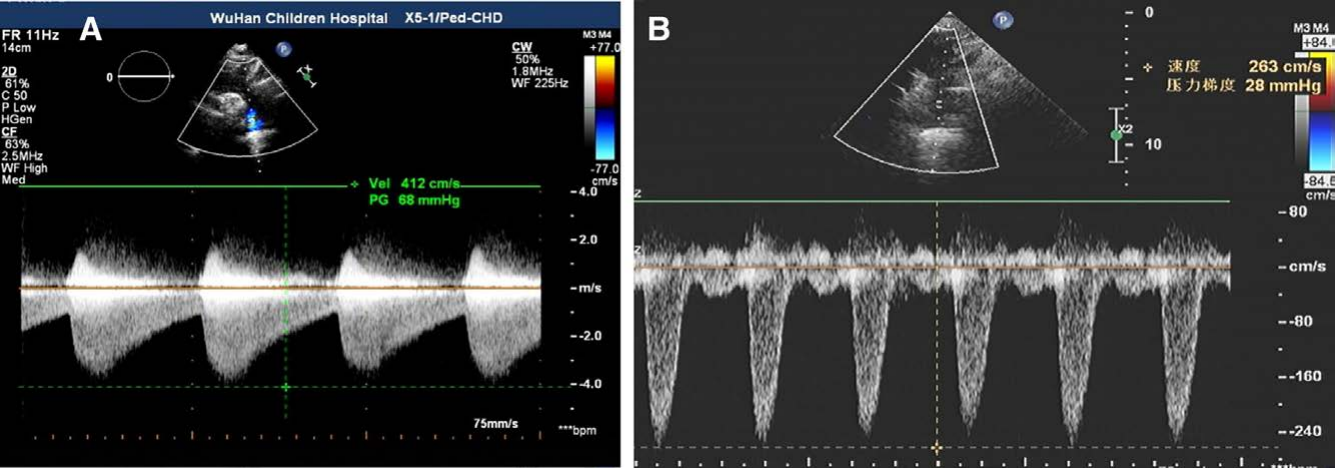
Pulsed Doppler assessment of blood flow velocity in CoA. (A) Pulse Doppler ultrasound at the site of CoA before surgery showed an acceleration of flow up to 4.12 m/s. (B) Postoperative pulsed Doppler ultrasound of the CoA site showed a decrease in blood flow velocity to 2.63 m/s. The 2D cardiac ultrasound data were as follows: EDV 71 mL, ESV 22 mL, SV 49 mL, EF (%) 69%, LVIDd 40.32 mm, LVIDs 24.76 mm, LVPW 7.5 mm, and IVS 10 mm. The morphology and structure of the heart valves were normal, and no significant regurgitation was detected. CoA = coarctation of the aorta, EDV = end-diastolic volume, EF = ejection fraction, ESV = end-systolic volume, IVS = interventricular septum, LVIDd = left ventricular internal diameter diastolic, LVIDs = left ventricular inner diameter systolic, LVPW = left ventricular posterior wall, SV = systolic volume.
